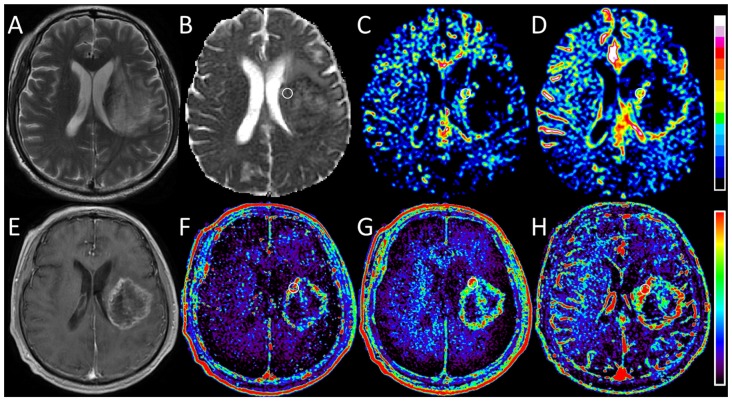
Images obtained in a 56-year-old man with glioblastoma (WHO grade IV). (A) T2-weighted image shows a heterogeneous hyperintense lesion in the left hemisphere. (B) ADC_0,1000_ map shows markedly decreased ADC value in the lesion. (C,D) IVIM *f* and SPF maps show increased *f* and SPF values in the corresponding area of the contrast-enhanced lesion as shown in (E). (E) On contrast-enhanced T1-weighted image, a rim-enhanced mass with central necrotic changes is seen correspondingly. (F–H) DCE MR imaging parametric maps of K^trans^, v_e_ and v_p_ show markedly increased values in the corresponding area of the contrast-enhanced lesion. When focusing on *f* and SPF maps, we can notice that the tumoral hyperperfusion area on SPF map is more visually obvious and more similar to that on DCE maps. Round-shaped regions of interest are marked on parametric maps.
